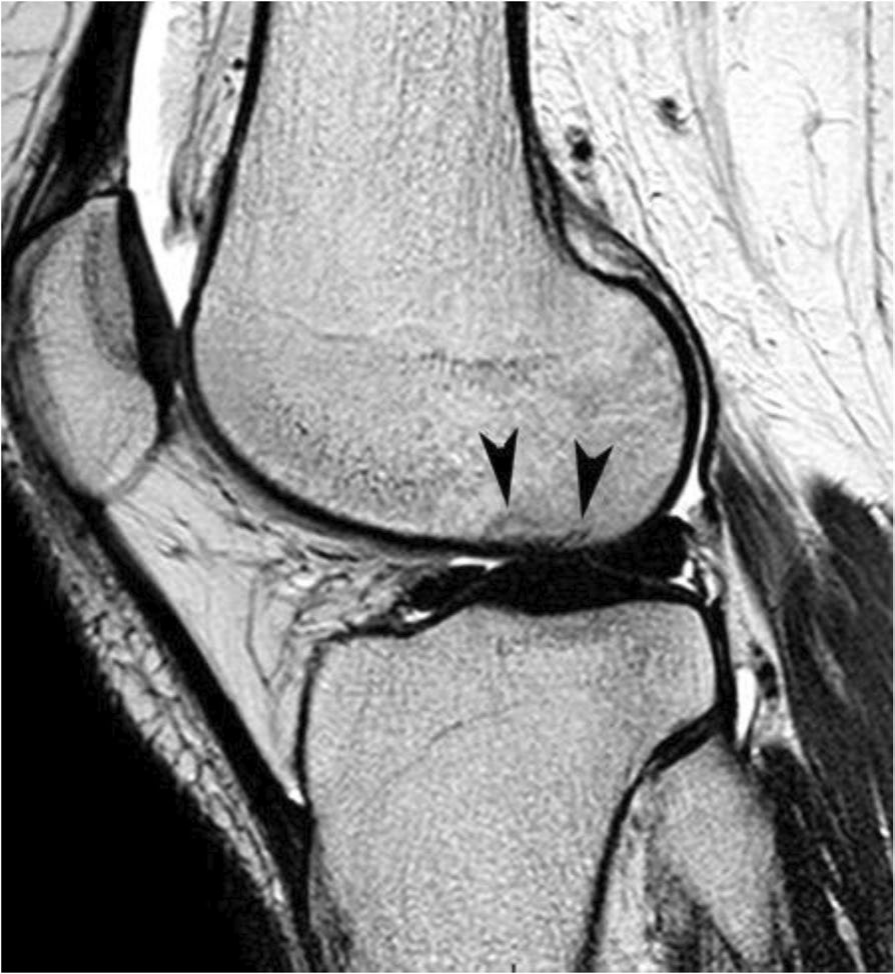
Trabecular fractures in case of CRPS1 (same MR examination as the previous figure). T2-weighted sagittal MR image shows thin, incomplete low-intensity linear images (arrows), as in a subchondral insufficiency fracture
